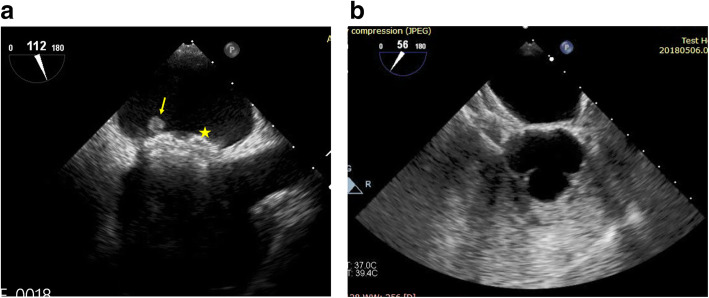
a 2D trans-esophageal echocardiography in bicaval view showing device in place (asterisk) and attached thrombus to the left atrial disc (arrow). b 2D trans-esophageal echocardiography in short axis mid-esophageal view showing device in place with bubbles filling the right side and no bubbles detected in the left side
